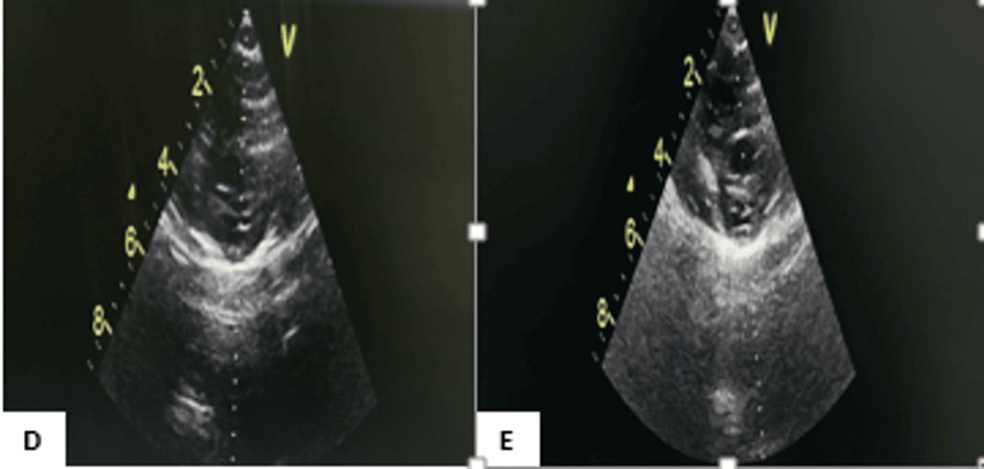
Cardiac ultrasound image of our patient showing initial improvement. D: Short-axis parasternal section showing posterior wall thickness at 4 mm; E: short-axis parasternal section showing left ventricular hypertrophy with ejection fraction at 66%
